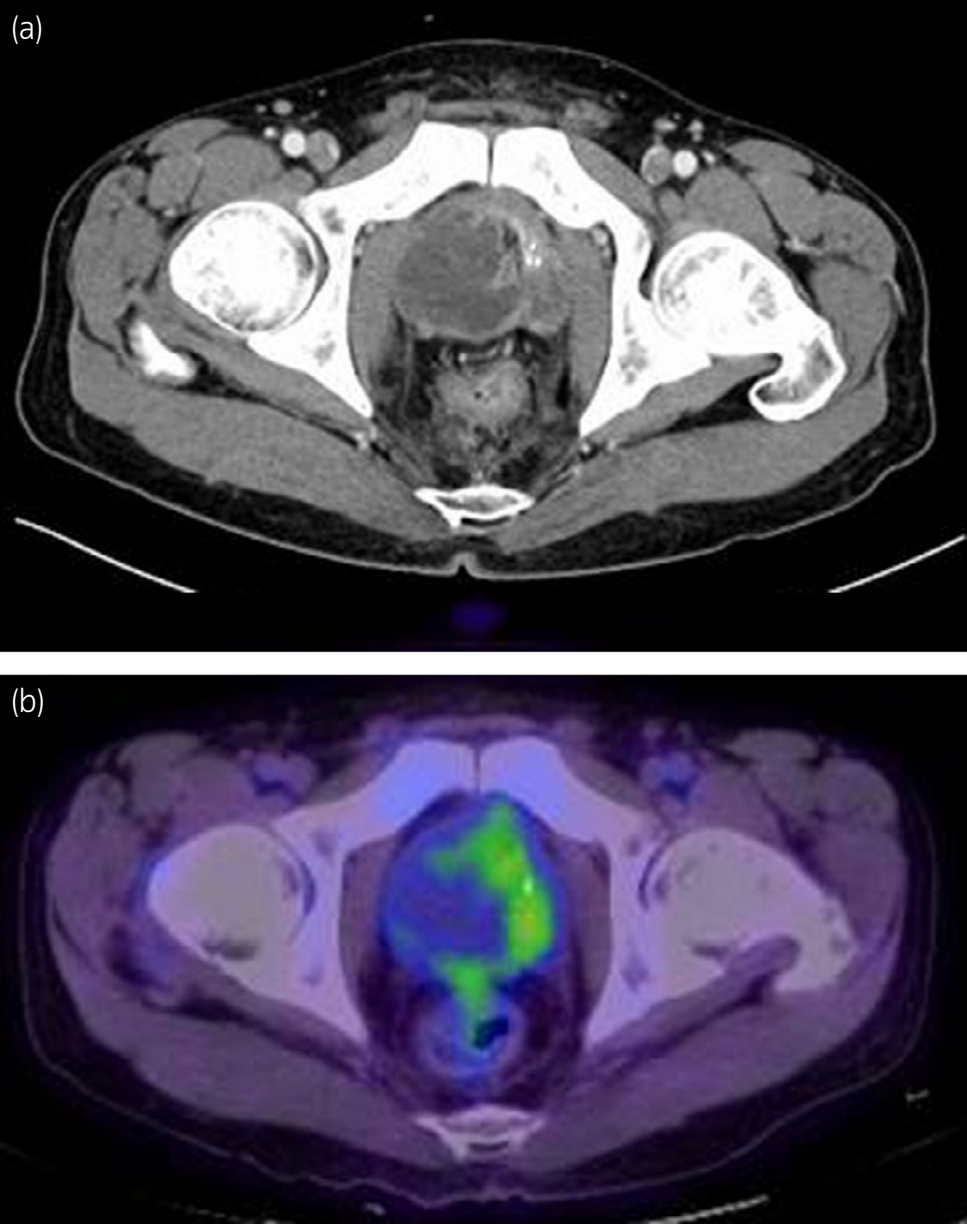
Contrast‐enhanced CT and PET‐CT images of the pelvis at diagnosis. (a) Transverse section, CT: A mucus‐enriched prostate tumor was detected with slight contrast at the edge. (b) Transverse section, PET‐CT: The prostate tumor showed an accumulation of FDG at the contrast‐enhanced site of the CT image.
